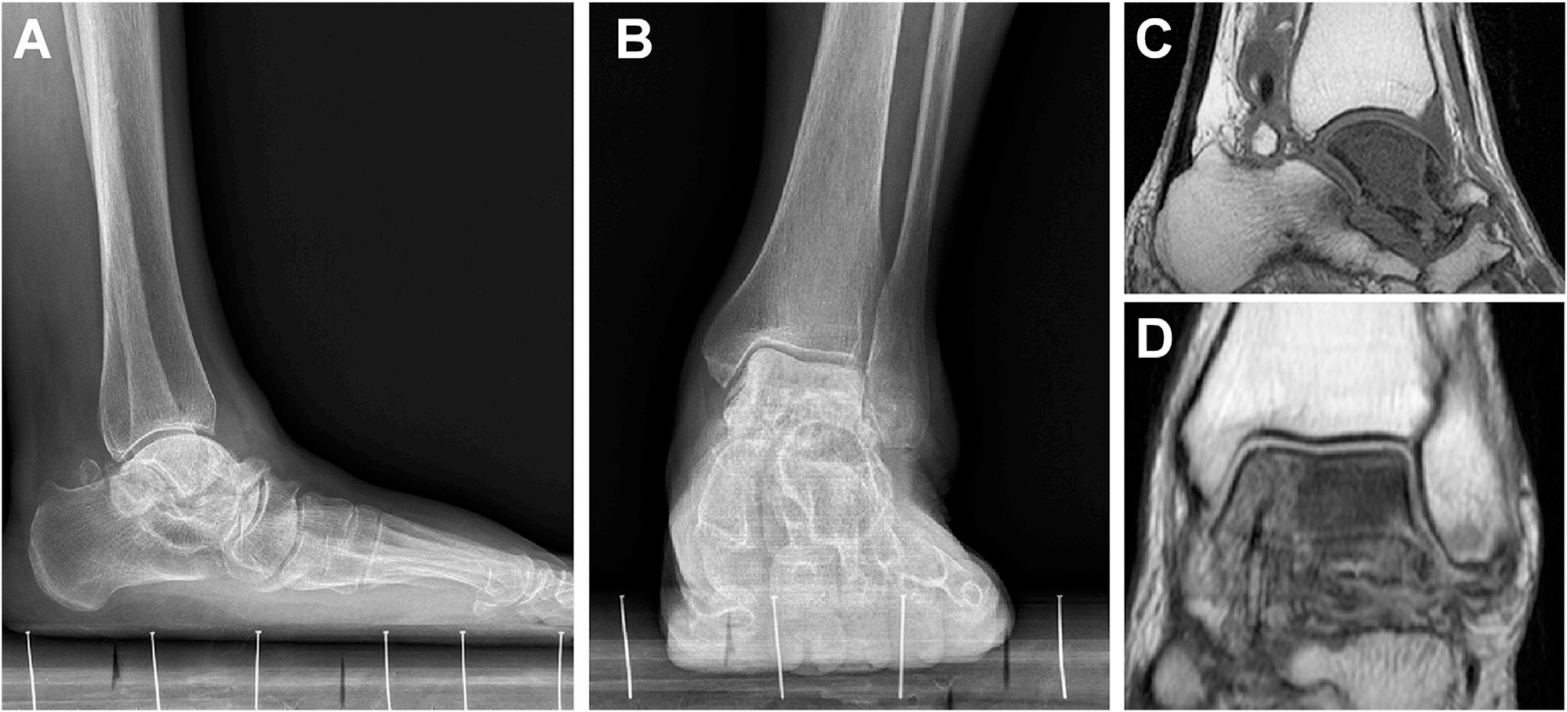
Preoperative weight-bearing anteroposterior and lateral views of patient 1 (A,B). TAN was confirmed by magnetic resonance imaging (C,D).
